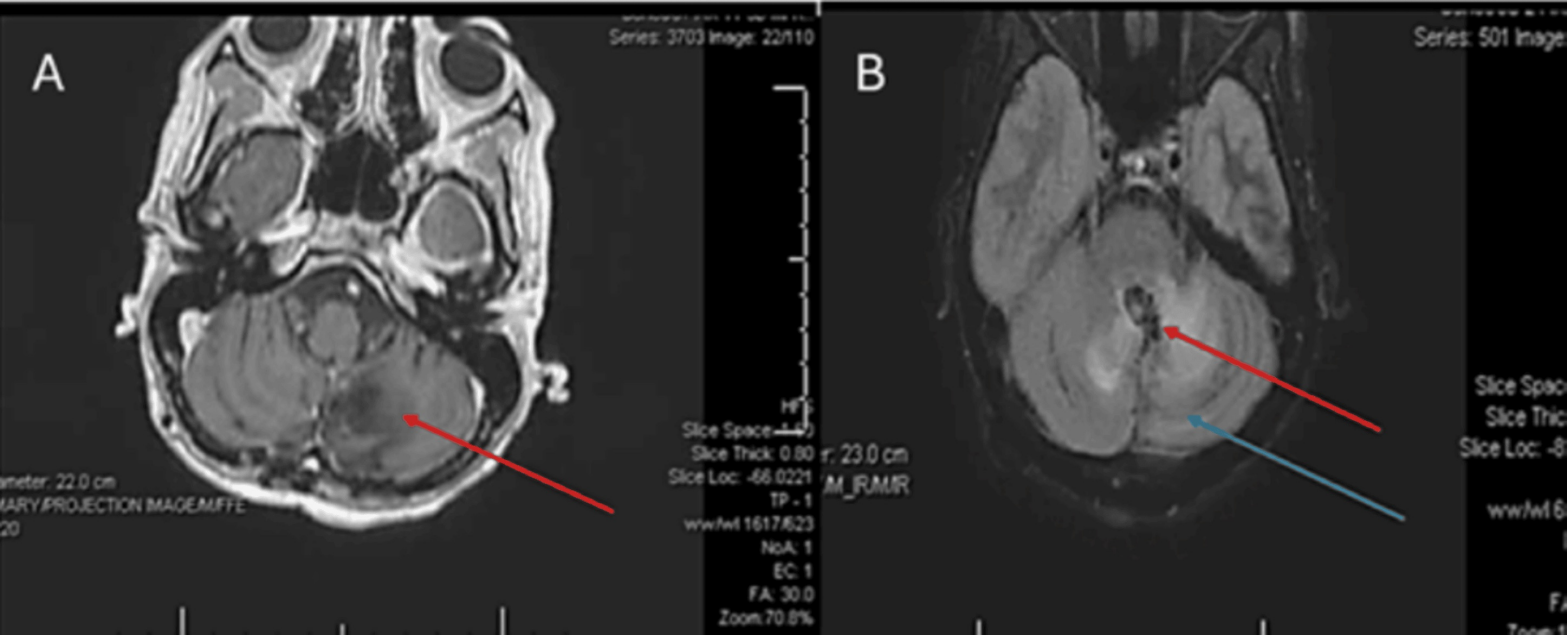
Contrast-enhanced axial T1-weighted sequence of the cerebellum (A) and corresponding T1 FLAIR sequence (B). The red arrows demonstrate expected post-surgical changes from the patient’s prior tumor removal. However, there is a notable absence of leptomeningeal enhancement in the cerebellar folia (blue arrow), which would be unusual in the setting of LMD. This further supports a diagnosis of SMART syndrome. LMD, leptomeningeal disease
